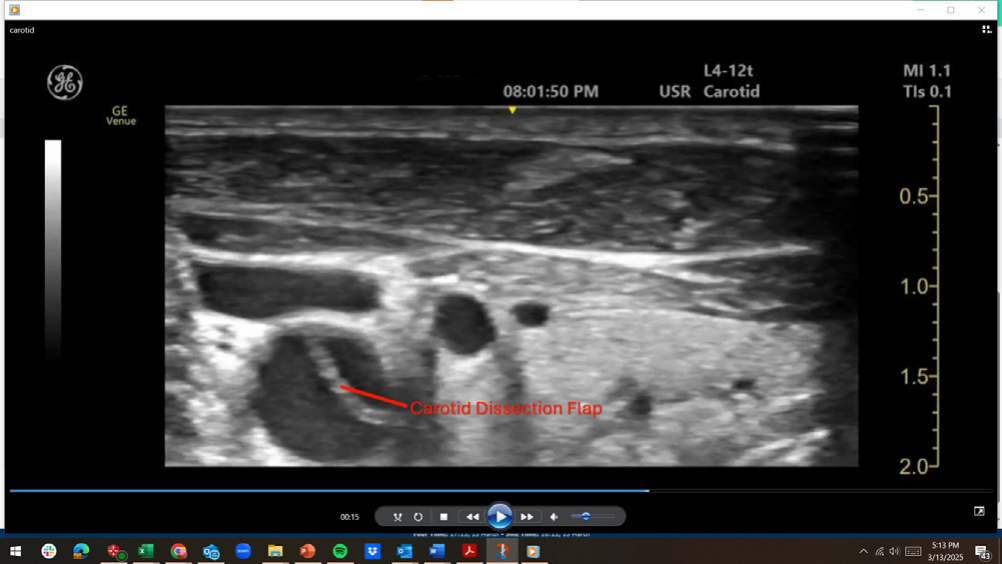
Transverse-axis point-of-care ultrasound of the right neck demonstrating carotid dissection.
